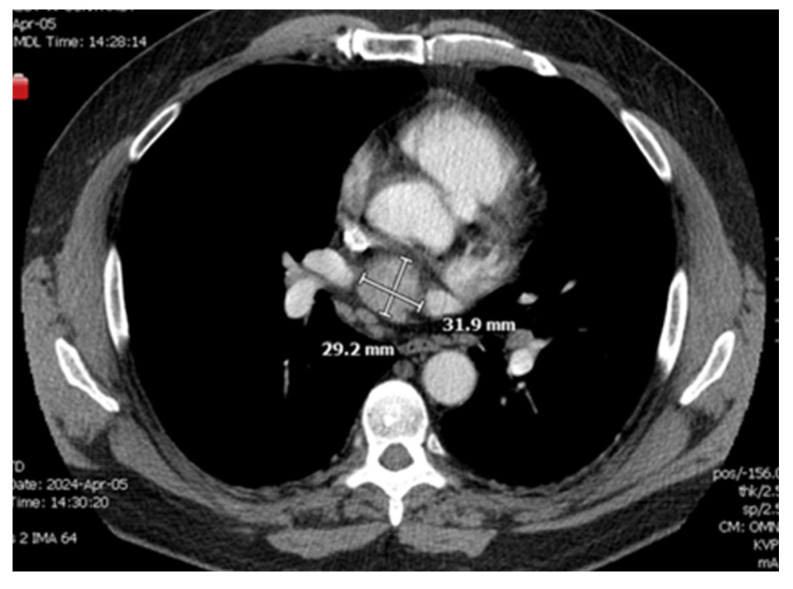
Re-demonstration of mediastinal mass in Axial CT scan.
